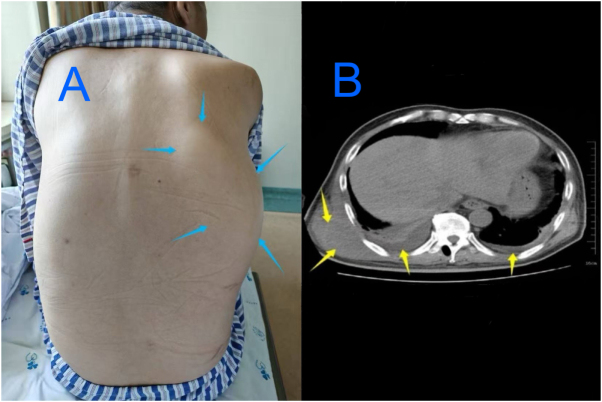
Images of the chest wall abscess and pleural effusion. (A) Photograph of the right chest wall abscess. The blue arrows indicate the margins. (B) Chest computed tomography scan showing a soft tissue mass in the right chest wall and bilateral small pleural effusions, more marked on the right side (yellow arrows).
